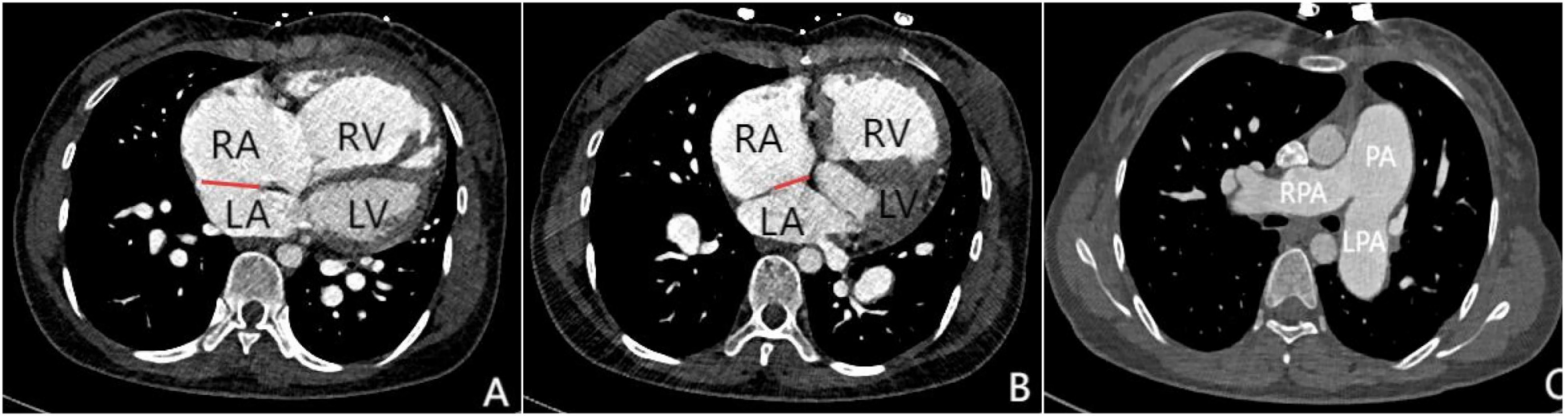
Panels (A–C) are all axial images from contrast-enhanced CT scans. Panels (A and B) demonstrate atrial septal defects at different locations (indicated by the red lines), measuring 3.1 cm and 1.8 cm in size respectively. Simultaneously, significant enlargement of the right atrium and right ventricle can be observed. Panel (C) shows marked dilation of the pulmonary trunk and both left and right pulmonary arteries, which is consistent with signs of pulmonary hypertension.
